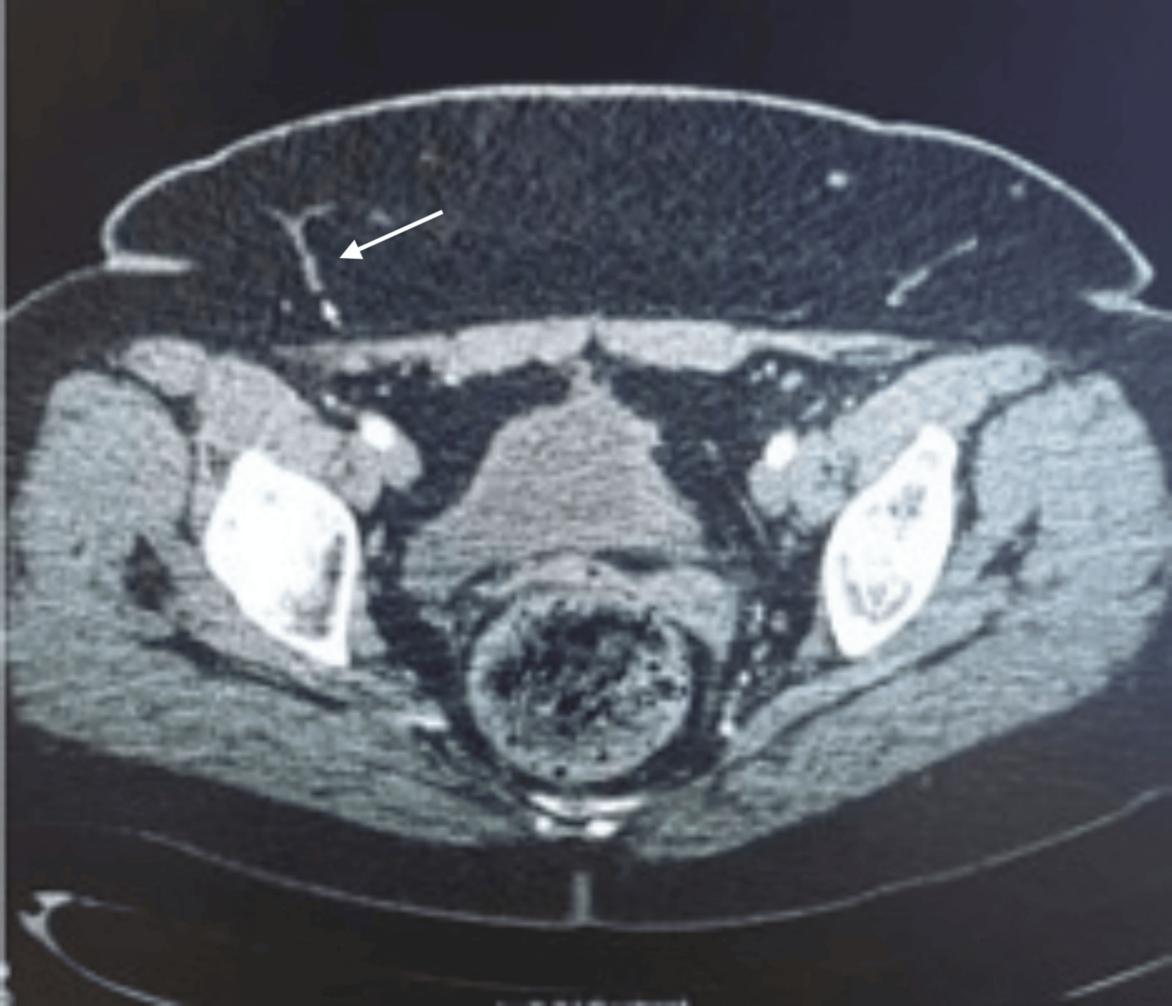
Preoperative CT angiogram (axial view) showing superficial system dominance
